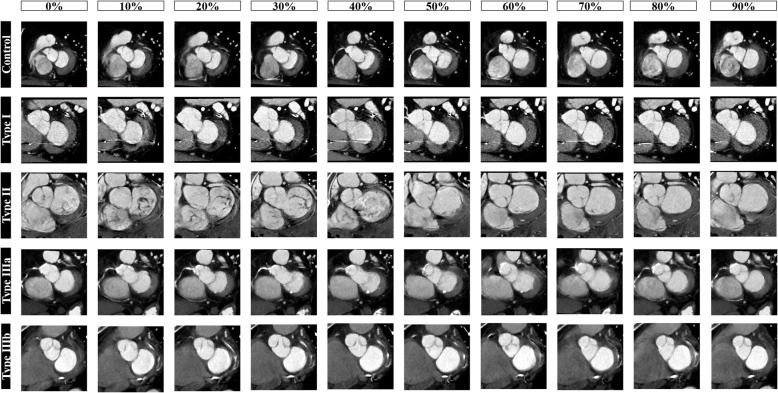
Short-axis reformatted cardiac computed tomography angiography images of the mitral valve annulus in each cardiac phase in exemplifying control patient and in mitral regurgitation patients according to Carpentier classification
